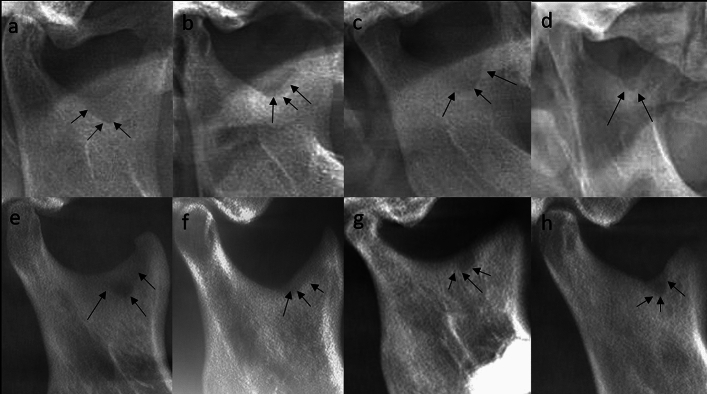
The cropped panoramic image of the MSD (a showing tear-drop shape, b showing semilunar shape, c showing circular shaped, d showing triangular shape; The cropped CBCT panoramic image of the MSD (e showing tear-drop shape, f showing semilunar shape, g showing circular shape, h showing triangular shape).
